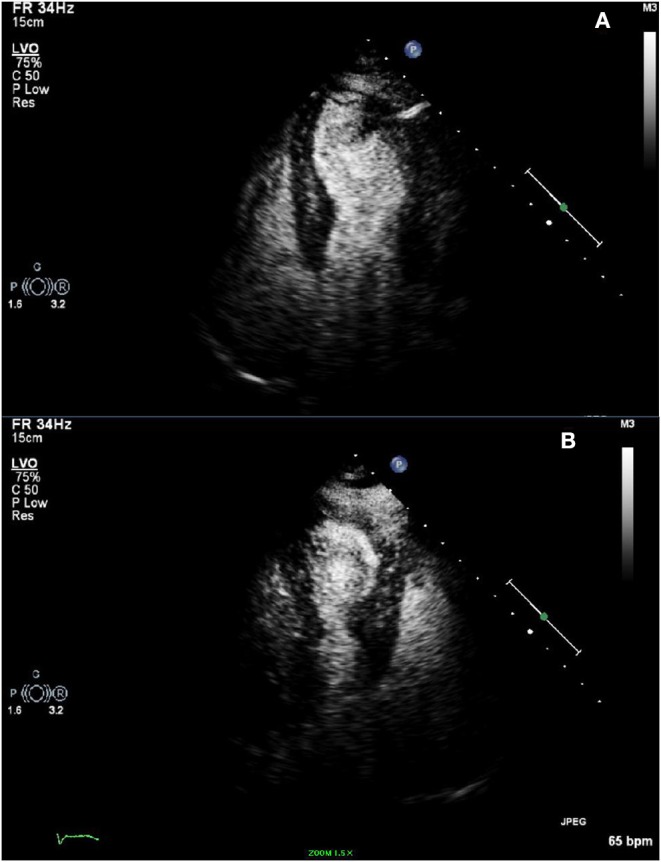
(A,B) Transthoracic echocardiogram with definity contrast demonstrating hypercontractility of the basal wall and akinesis of the mid to distal anteroseptal wall, anterior wall, mid to distal inferior and inferolateral walls, and apex.
